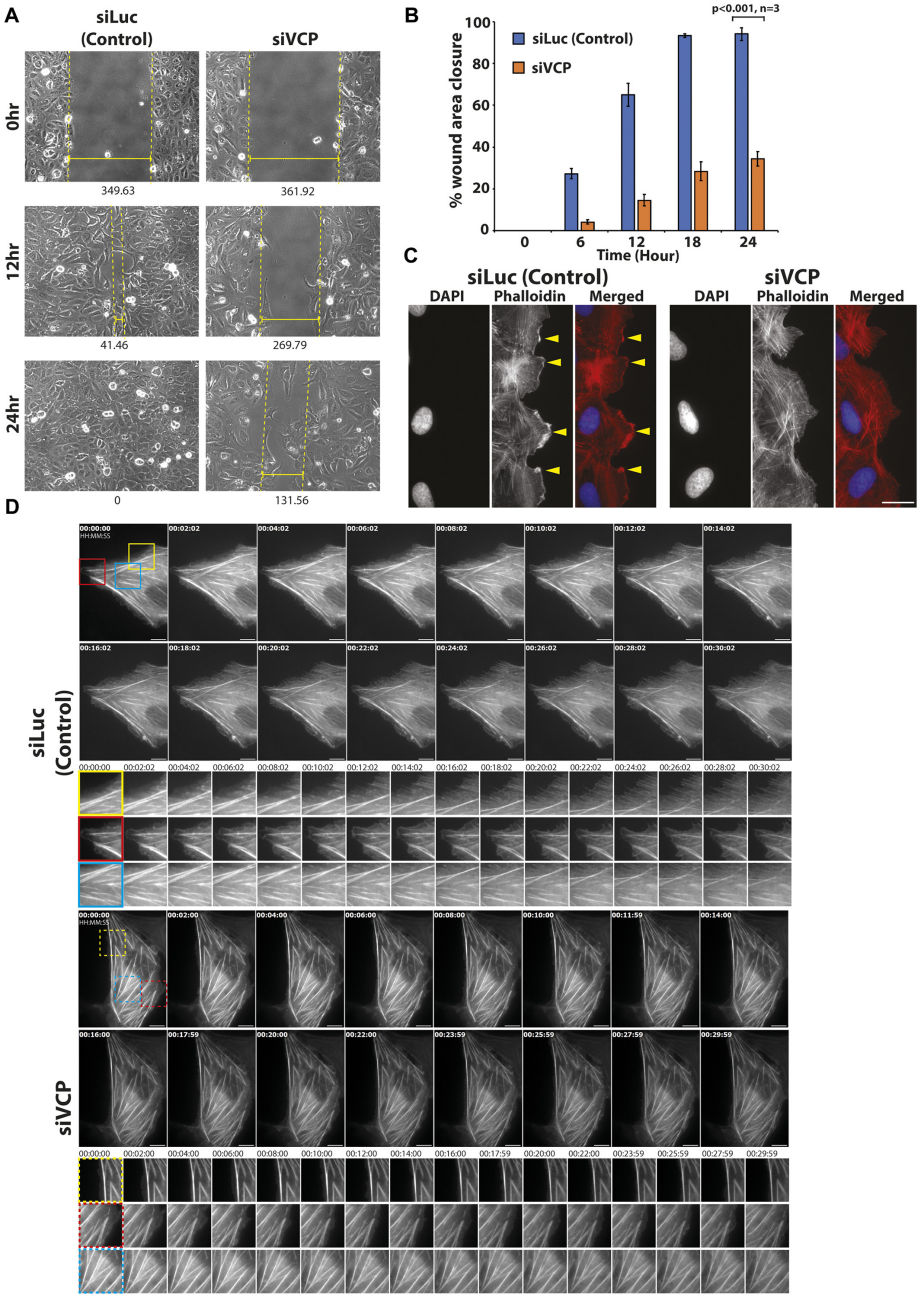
Knockdown of p97/VCP impaired proper cell migration. (A) Migration abilities of U-2 OS cells were observed in a scratch wound assay over a time course of 24 hours. Glass bottom dishes were scratched, and time-lapse images were taken over a time course. After 12 hours upon wounding, siLuc-treated cells have migrated efficiently towards the site of wounding. At 24 hours, the wound has fully healed. In siVCP-treated cells, migration was impaired, and minimal wound healing was observed. Numbers below images represent the width (nm) of the wound, and each image was obtained using Axiovision. Images were taken using 20× Phase contrast objective. (B) Scratch wound assay was analyzed by Image J (MRI wound healing tool plugin). Histogram shows the percentage of wound closure in the time-lapse experiments (*n* = 3 from 3 independent experiments, error bars show ± SEM). (C) In control U-2 OS cells, there was distinctive formation of lamellipodia at the leading edge of migrating cells (yellow arrowheads). Thin actin filaments were also observed. In siVCP knockdown cells, there was no clear formation of lamellipodia in migrating cells. (D) Live cell-imaging of control and siVCP knockdown U-2 OS cells showing the difference in actin dynamics in the presence and absence of p97/VCP. In control cells, actin filament bundles are dynamic while in siVCP knockdown cells, most filament bundles were static over the course of the time-lapse. Scale bar = 10 µm. Color boxes are enlarged images of the movie.
